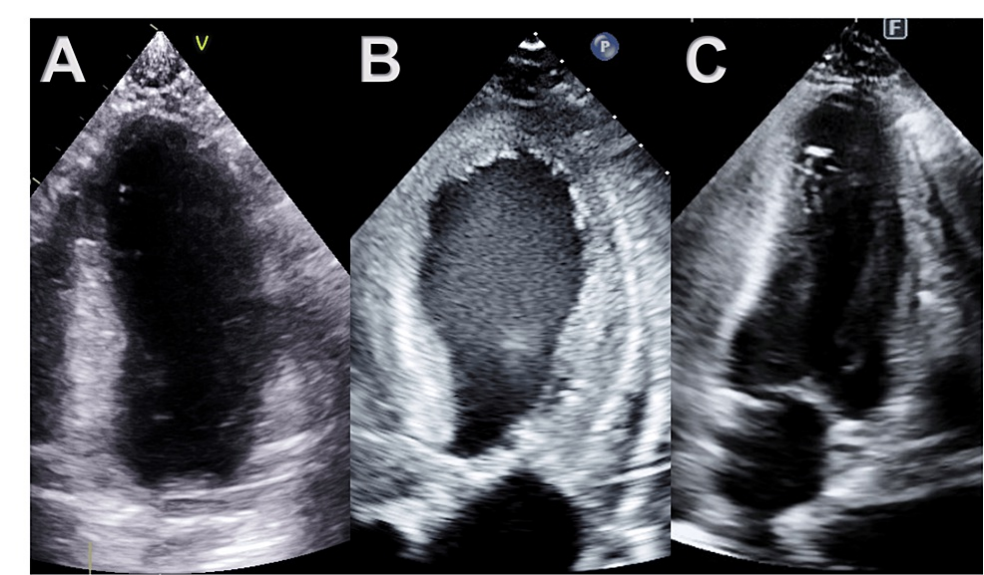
Echocardiography images (apical 2-chamber views) (A) Normal apical systole 4 months pre-op, (B) mid and distal anterior septum and entire apex are akinetic with preserved contraction in basal portion at six days post-OLT, (C) Recovered LV function with normal apical systole 20 days post-OLT OLT: orthotopic liver transplantation; LV: left ventricle
